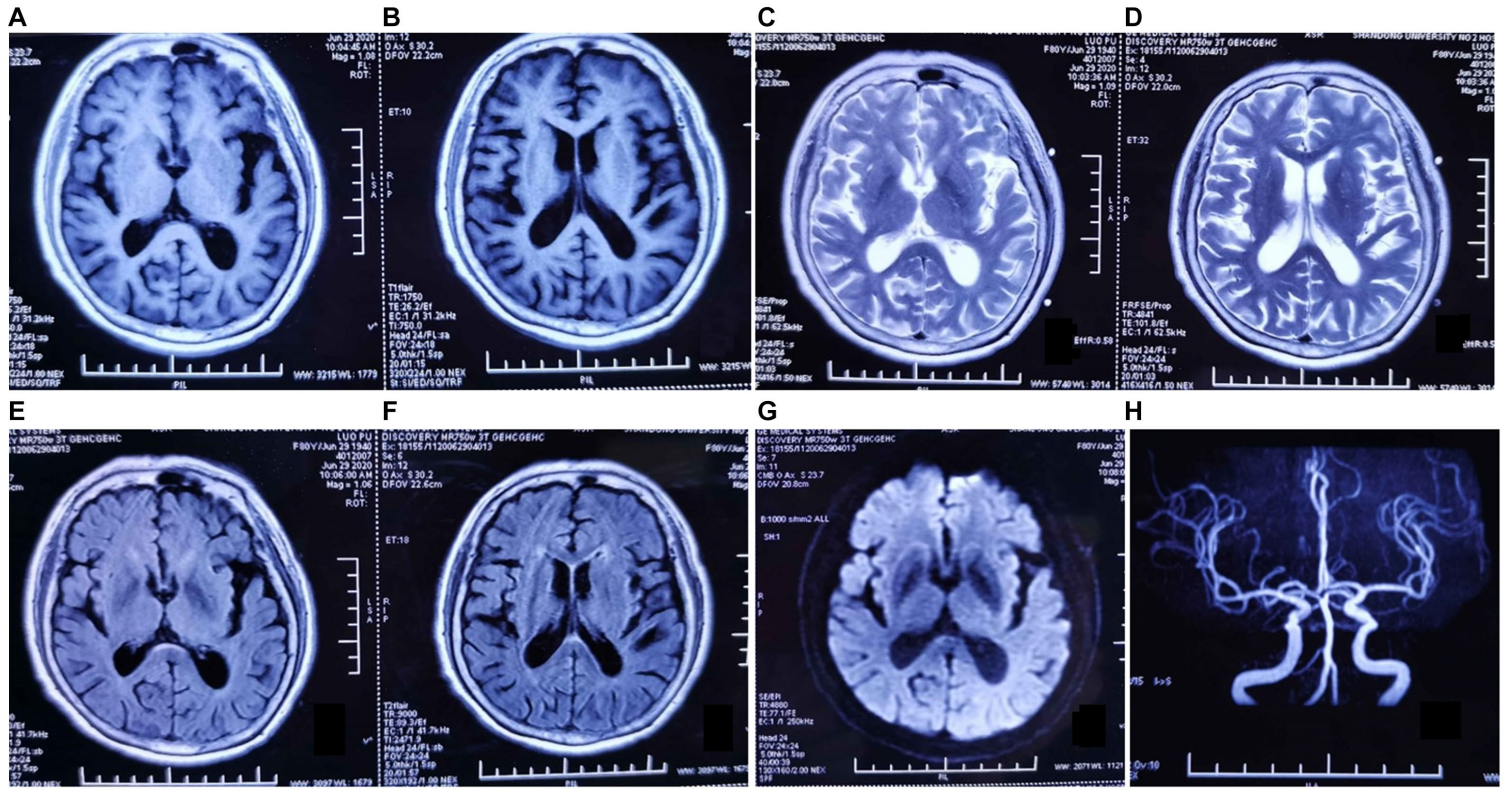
Magnetic resonance (MR) imaging findings dated June 2020. (A–G) Axial T1-weighted, T2-weighted, and fluid-attenuated inversion recovery MR images show mild demyelinating changes in the anterior horn of the lateral ventricle, abnormal punctate signals in the posterior limb of the internal capsule, and thickening of the cerebral dura mater. (H) MR angiography image showing no abnormalities in the large intracranial arteries.
